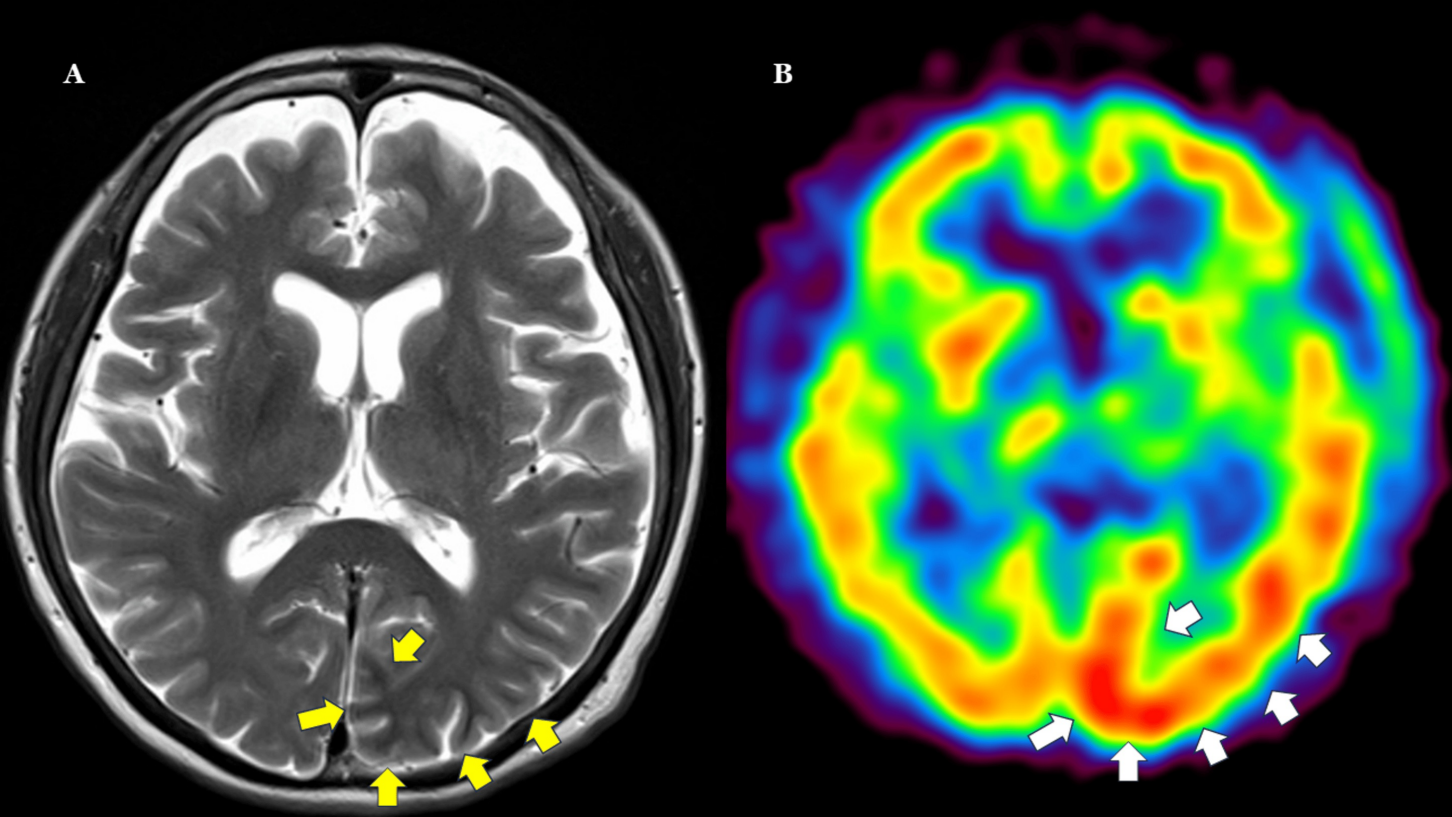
¹²³I-IMP SPECT on day 10 of right homonymous hemianopia onset in comparison with T2-weighted MRI on day 7. On ¹²³I-IMP SPECT (B), increased accumulation is observed in the cortical region of the left occipital lobe (white arrow), corresponding to the hypointense area (yellow arrow) on T2WI MRI (A) obtained on Day 7. ¹²³I-IMP SPECT: N-isopropyl-p-[¹²³I]iodoamphetamine single-photon emission computed tomography
